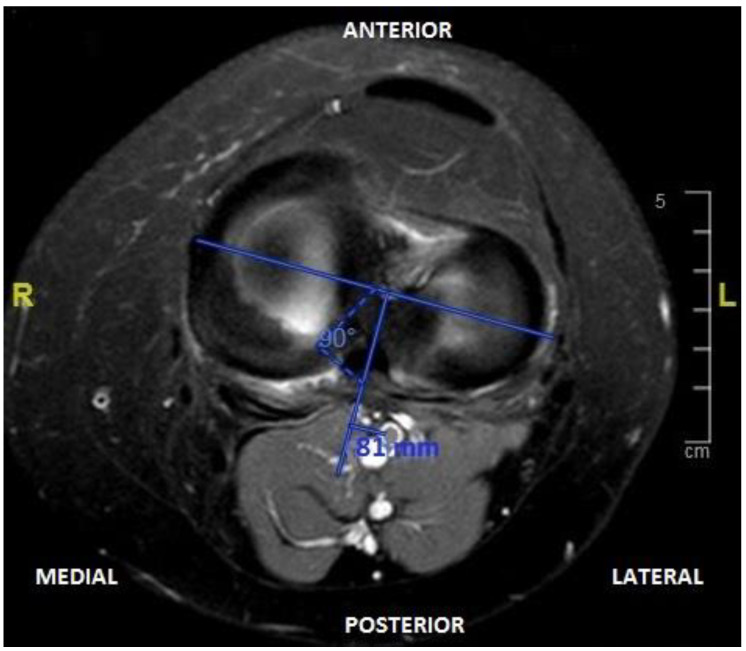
Axial section of the left knee in magnetic resonance imaging, where we observe the determination of the midline drawn from the center of the tibial spine at 90° from the transverse axis of the knee and distance from the popliteal artery to said line (IM–PA).
